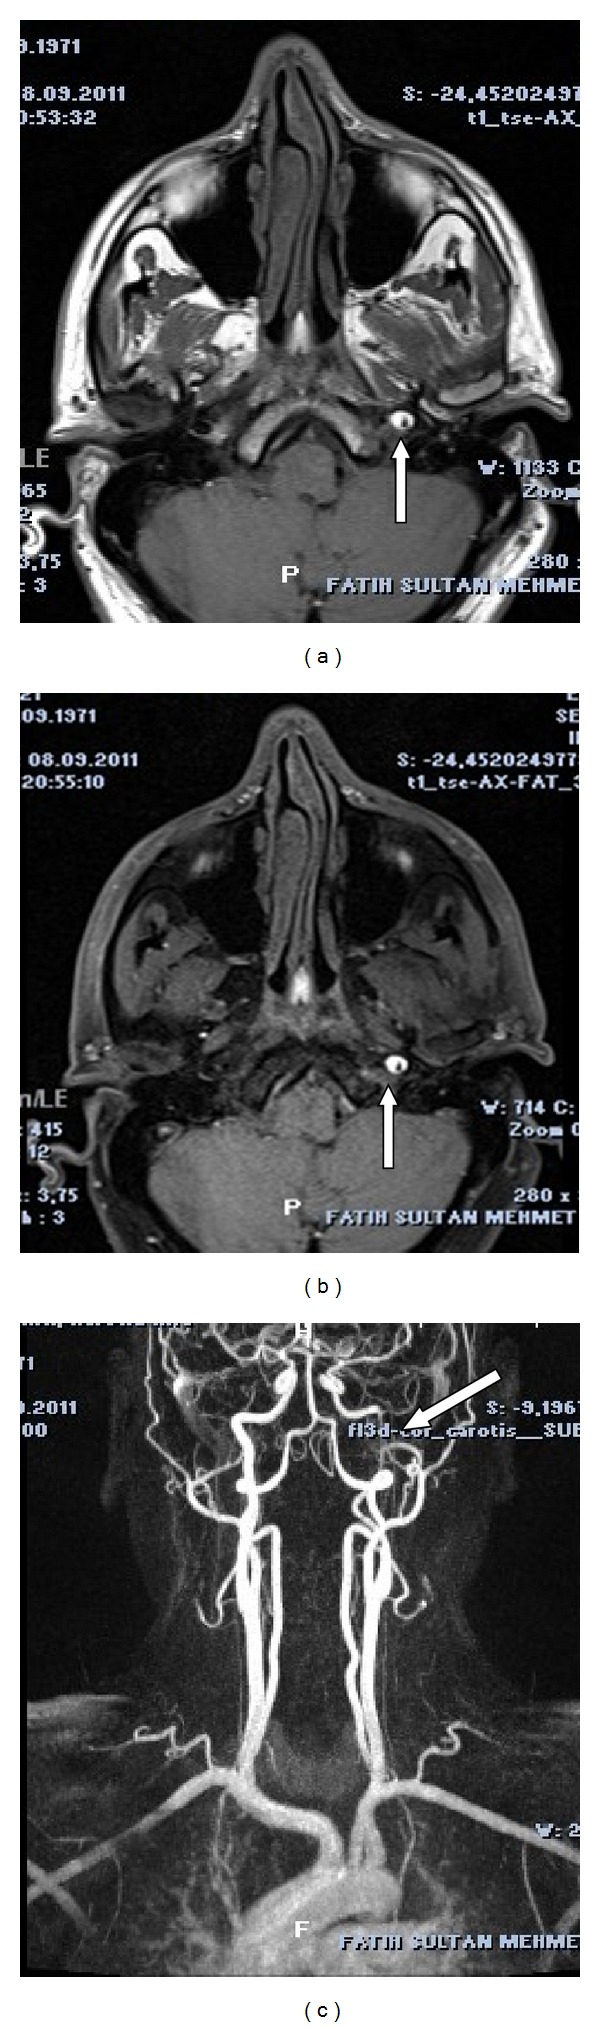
(a) Hyperintense area surrounding the left ICA on T1-weighted axial cranial MRI (white arrow). (b) Intramural hematoma on the left ICA on T1-weighted, fat-suppressed axial cranial MRI (white arrow). (c) Marked decrease in blood flow within cervical and temporal petrous segment of the left ICA on cervical MRA (white arrow).
